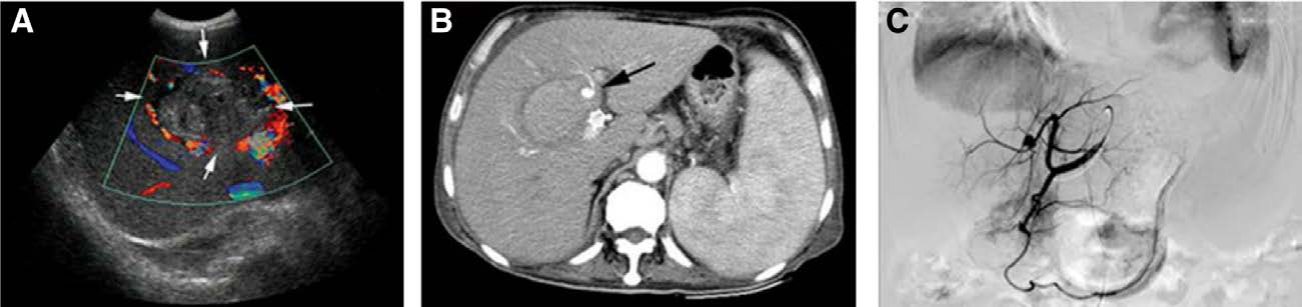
Images of the liver 3 days after TIPSS insertion. (A) Abdominal Doppler Ultrasonography showed a 8.6 × 5.4 cm hybrid echogenic mass in porta hepatis with no blood flow (white arrow). (B) Abdominal CT scan showed the presence of a 5.5 × 5.34 cm intrahepatic hematoma and a left hepatic pseudoaneurysm (black arrow). (C) Celiac angiography performed 48 h after onset of the hematoma and confirmed the hepatic pseudoaneurysm. The hepatic artery laceration began at the level of the branch of the left hepatic artery. The right hepatic artery which originated from superior mesenteric artery was normal.
